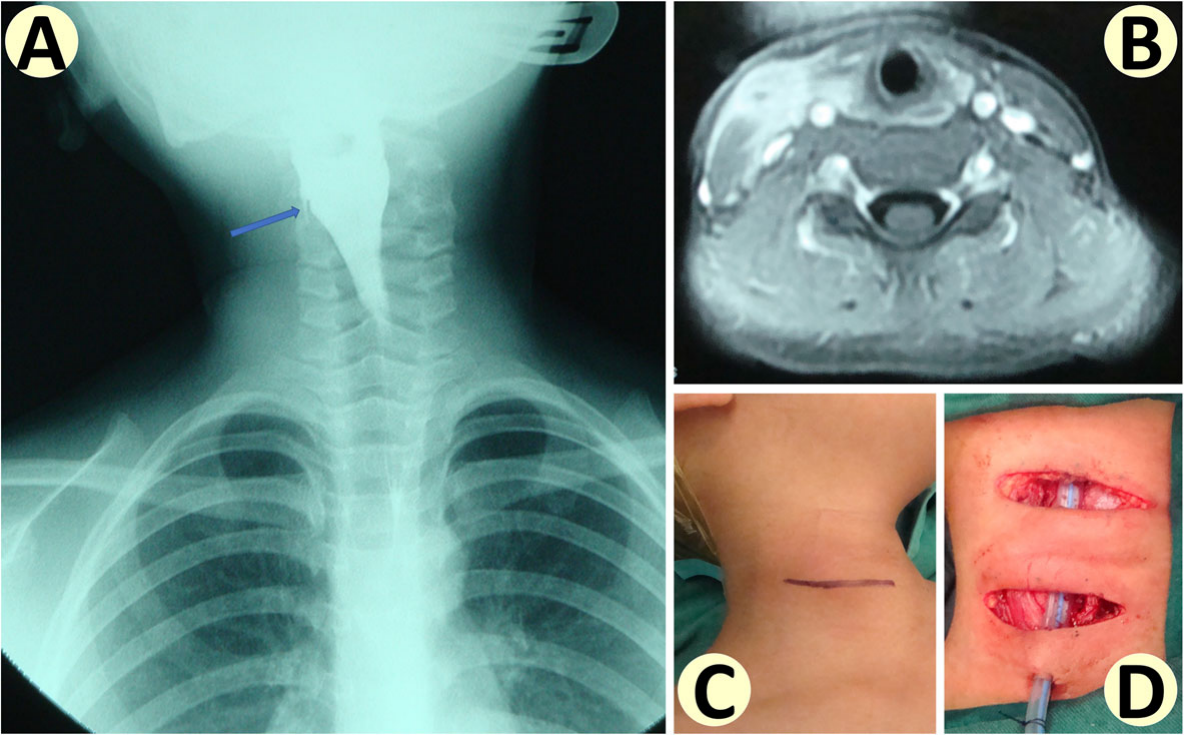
Right-sided fourth branchial pouch sinus. a Tiny amount of right-sided barium outflow from pyriform sinus can be seen on X-ray film (blue arrow). b Right-sided massive cervical inflammation of the same patient. c Pre-operative cervical photo shows no cervical cutaneous orifice. d Step ladder incision for this patient, with drainage tube in surgical field
